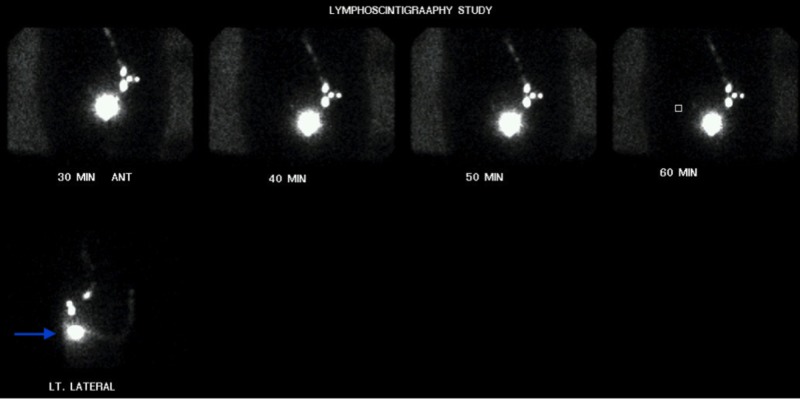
Lymphoscintigraphy study, delayed anterior and lateral views Delayed anterior and lateral emission and transmission images of the pelvic region revealing the anterior location of the sentinel node (arrow).
